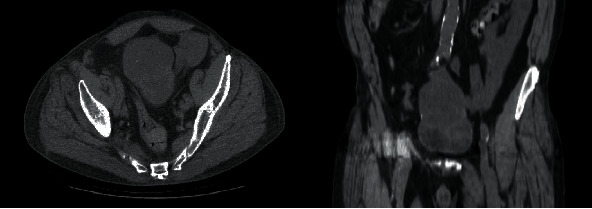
Axial and coronal cross-sectional imaging via CT abdomen and pelvis revealing an exophytic mass arising at dome of bladder.
